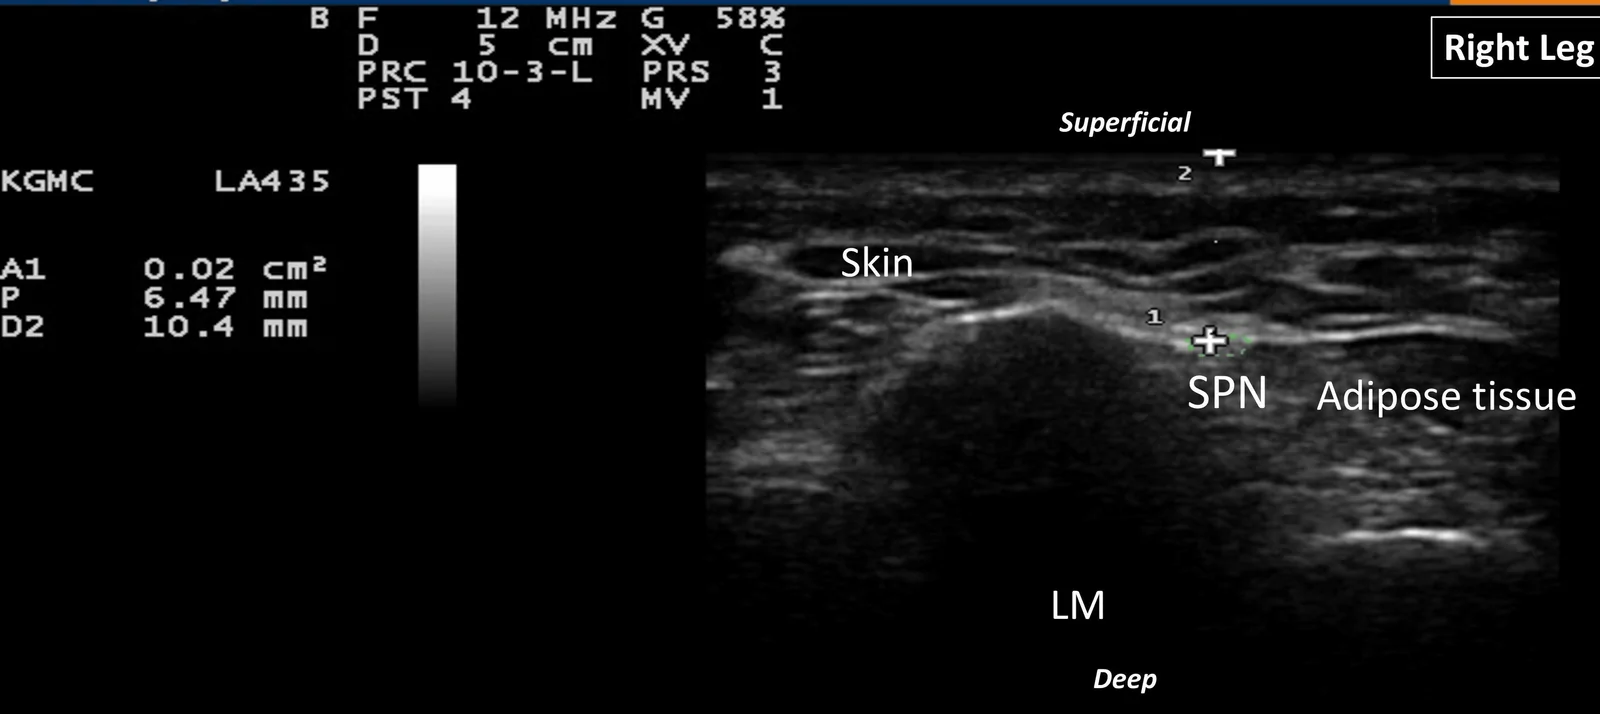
Ultrasonographic image of right leg showing the superficial peroneal nerve at 5 cm above the lateral malleolus. A, artery; f, fibula; LM, lateral malleolus; SPN, superficial peroneal nerve; t, tibia.
